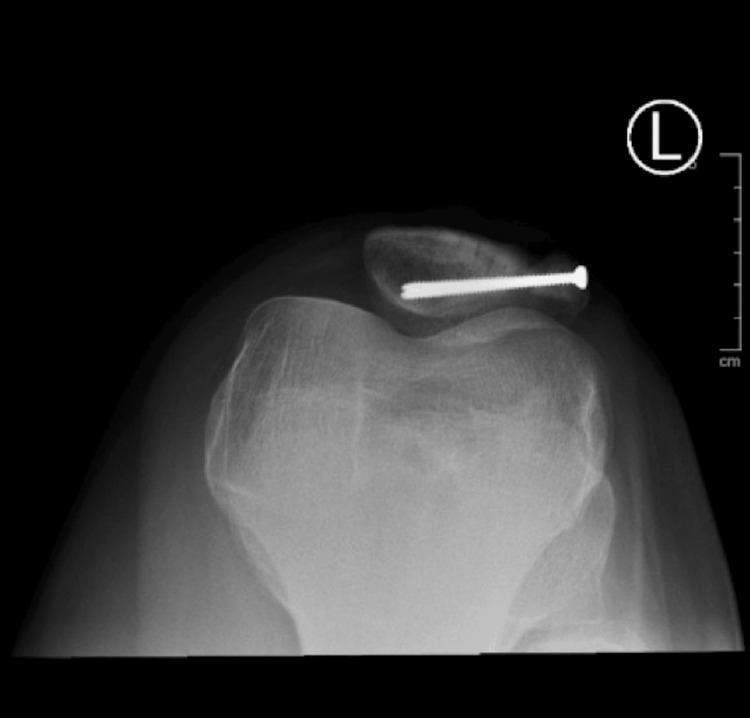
Six-month postoperative X-ray L knee sunrise view
